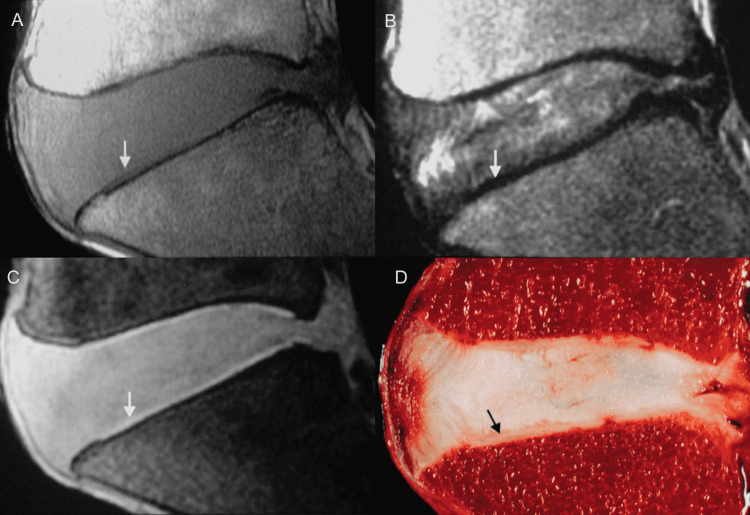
Cartilaginous end plates. (A) Sagittal T1-weighted MR image shows cartilaginous end plate (CP) (in portions) (arrow) with intermediate signal intensity. The deeper layer of CP investing subchondral bone is of low signal intensity owing to calcified cartilage, and differentiation between cartilage and bone is not possible. (B) Corresponding sagittal T2-weighted MR image shows cartilaginous end plate (CP) and subchondral bone of uniform, low signal intensity (arrow) compared to the intervertebral disc. (C) Fat-suppressed 3D-SPoiled Gradient-Recalled echo sequence (SPGR) MR image exhibits cartilaginous end plate (CP) as the distinct, continuous band-like structure of high signal intensity. (D) Corresponding sagittal anatomic section outlines the cartilaginous end plate (CP).
